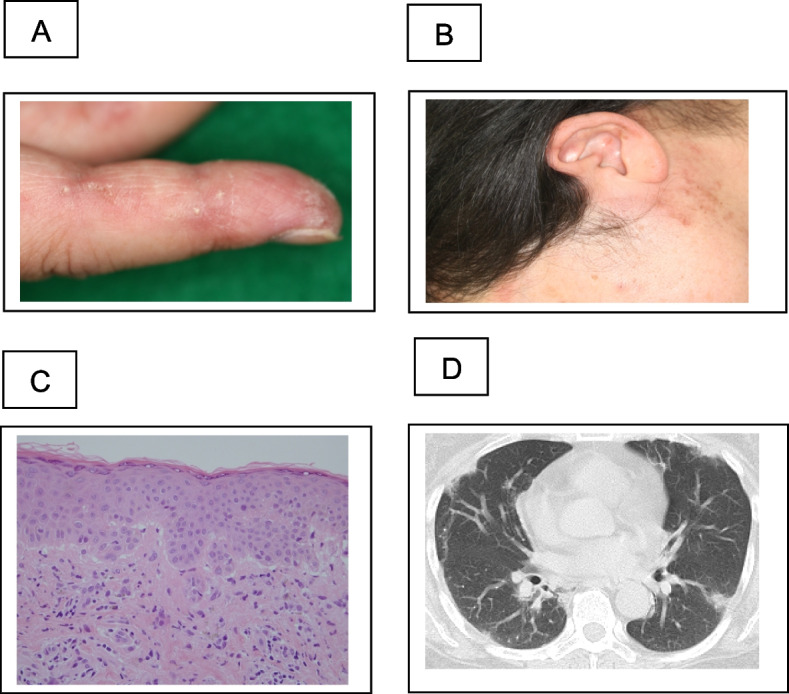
Characteristic findings of anti-MDA5 antibody-positive dermatomyositis. A Reverse Gottron’s sign and B exudative erythema, representing characteristic findings of anti-melanoma differentiation-associated gene 5 antibody-positive dermatomyositis. C Dermatopathological findings showed liquid degeneration of the epidermal basement membrane, dermal mucin deposition, and perivascular inflammatory cell infiltration of the dermis. D Computed tomography (CT) findings on first admission. Infiltrative and interstitial shadows are observed just below the pleura
